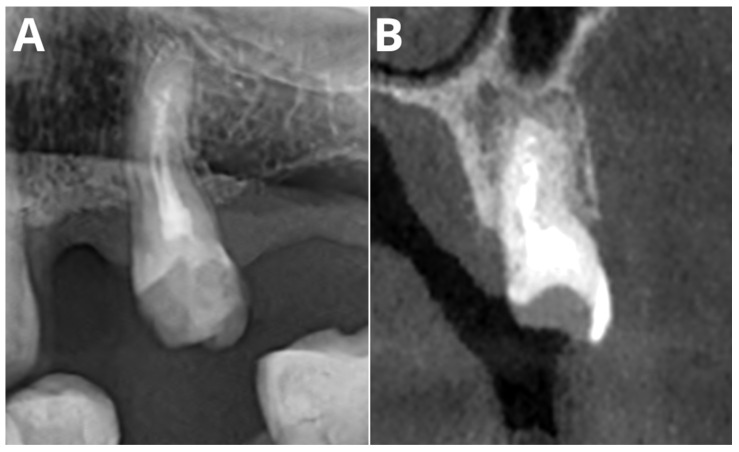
Sample false positive AI diagnosis of PL in OPG, correctly diagnosed by readers in OPG (A). No signs of periapical radiolucency in CBCT (B).
